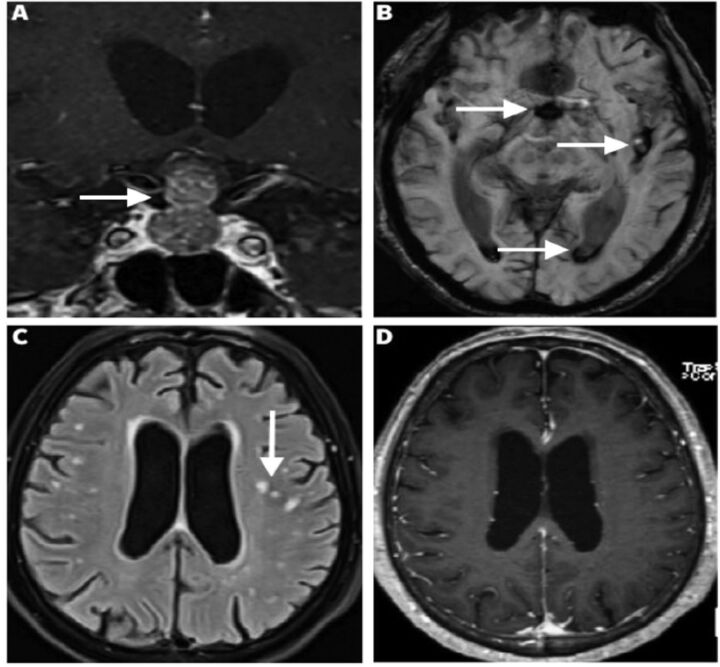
Brain magnetic resonance imaging showing: A) postcontrast coronal view demonstrating enhancing sellar mass with suprasellar extension giving the Snowman sign, typical for pituitary macroadenoma, there is also mild ventricular dilatation; B) susceptibility weighted imaging revealing blooming in the Sylvian fissure and sulci representing subarachnoid haemorrhage, in the posterior horns of the lateral ventricles representing intraventricular haemorrhage and in the suprasellar part of the pituitary macroadenoma in keeping with pituitary apoplexy; C) FLAIR; and D) postcontrast images demonstrating multiple white matter hyperintense foci without enhancement representing small vessel disease/infarctions.
